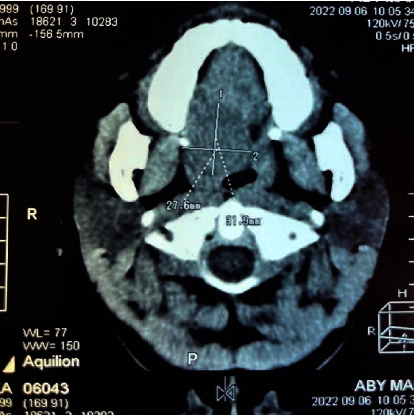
Axial-slice CT scan shows hypodense mass of the palate.
